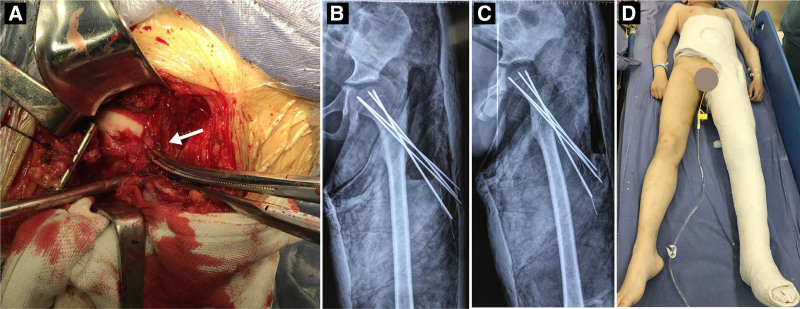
Imaging data during operation. (A) Open reduction and internal fixation using an anterolateral approach. The white arrow indicated the slipped capital femoral epiphysis. (B) Antero-posterior radiograph of the left hip. (C) Lateral radiograph of the left hip. (D) One-and-a-half hip spica cast.
